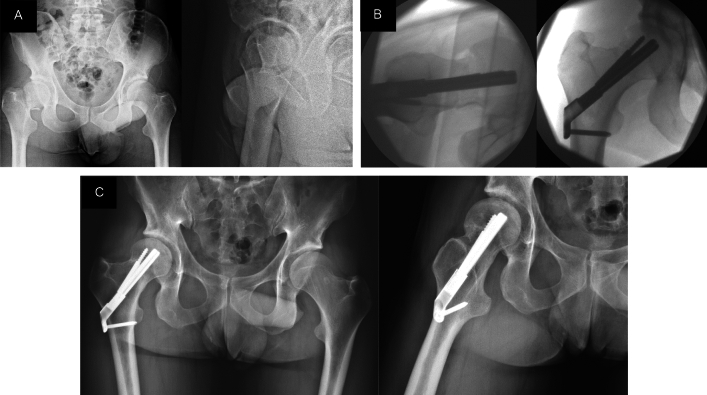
(A) AP and axial radiographs of the pelvis and right hip were taken in the emergency department of a 29-year-old patient who suffered a Garden III subcapital fracture and Pauwels III fracture of the right femur. (B) Intraoperative fluoroscopy controls with AP and axial views. Correct reduction of the fracture was observed in both the AP and axial projections. (C) AP and axial hip radiographs during the annual follow-up in the clinic. Complete consolidation of the fracture was observed.
